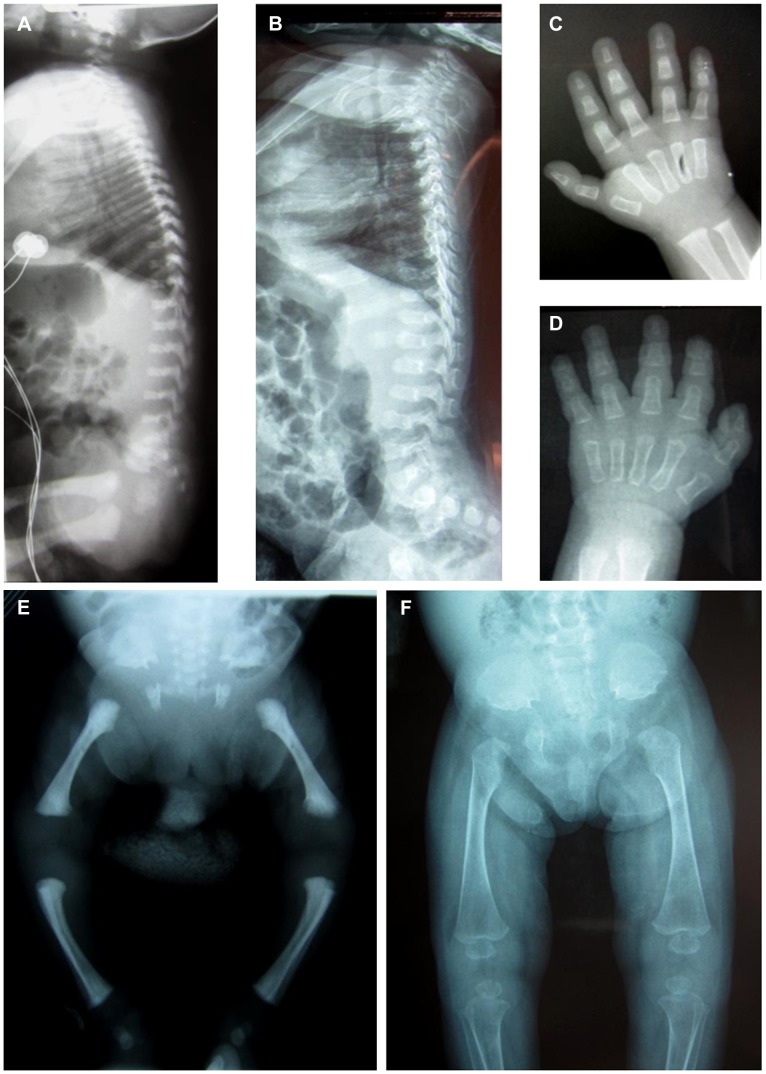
Radiological features of the patients F2-IV.3 and F1-IV.3. Radiographs of patient F2-IV.3 at 9 months (A, C, E) and F1-IV.3 at birth (B, F) and at 3 months (D) show platyspondyly, square iliac bones, and delayed epiphyseal ossification.
